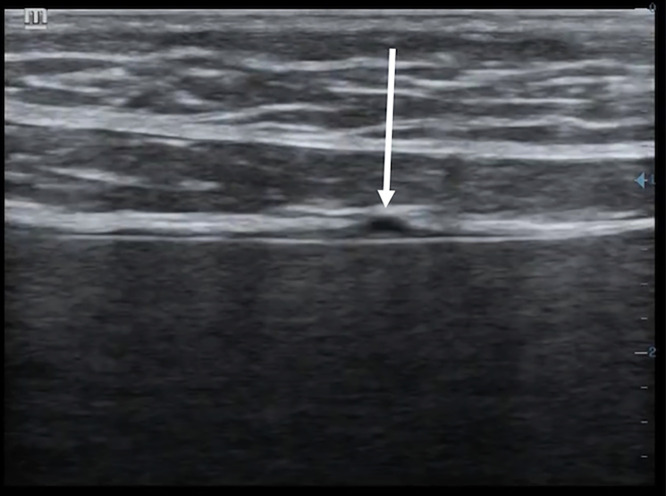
Ultrasound of the peritoneum superficial to the liver revealed multiple, hypoechoic <3-mm micronodules.
